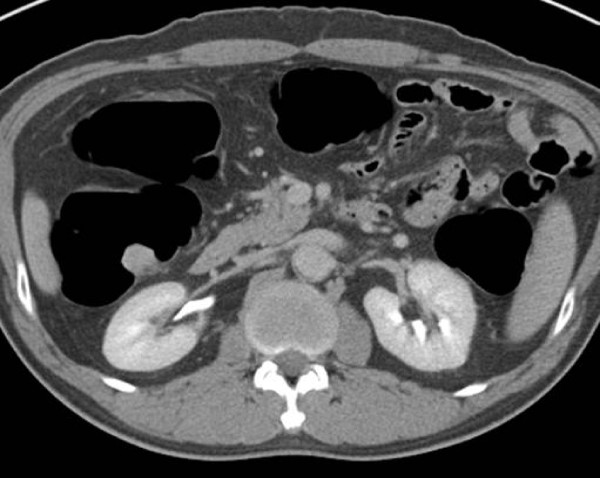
Homogeneous enhancement of mass on abdominopelvic CT.
